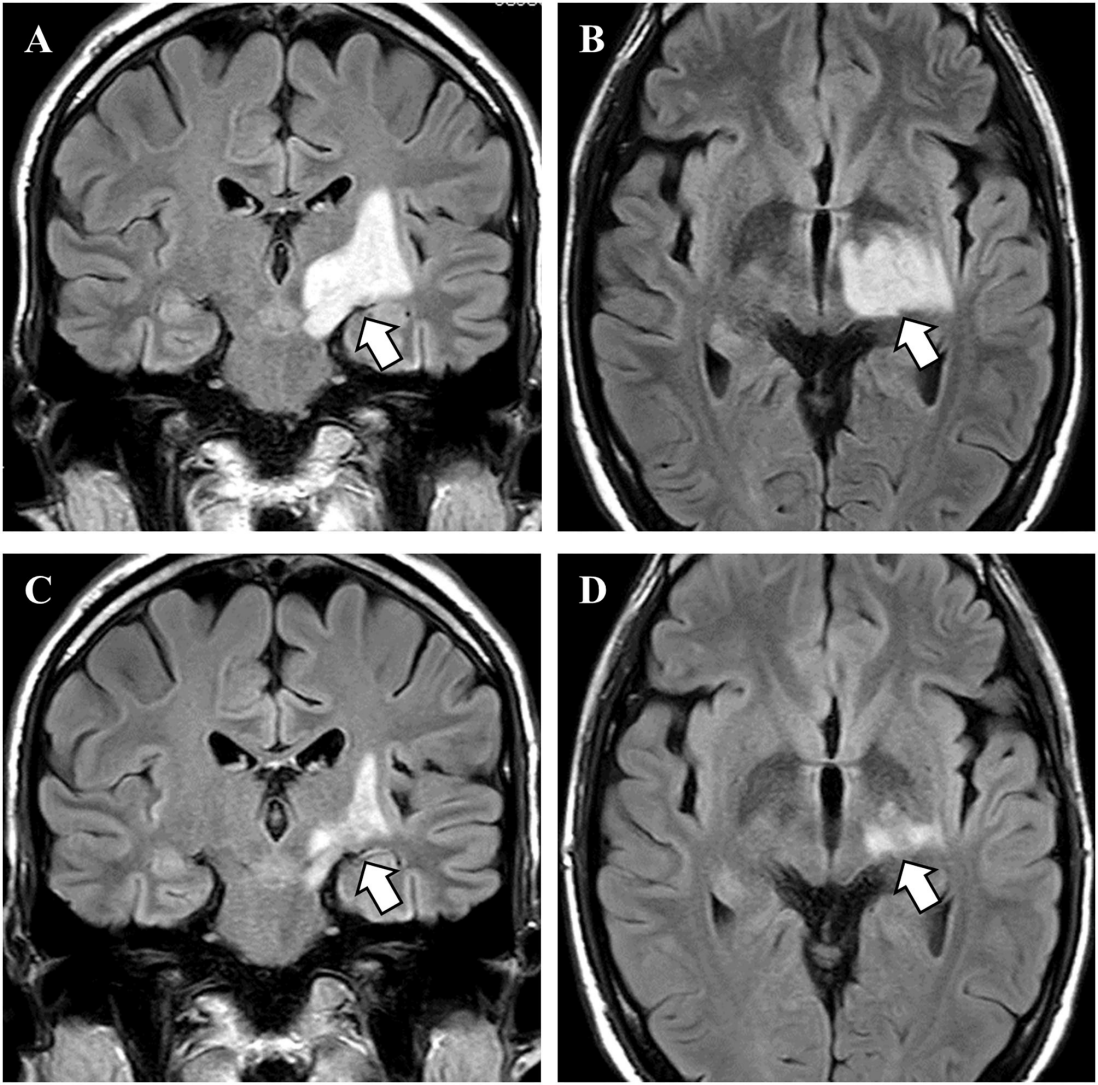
MRI findings before and after treatment. (A,B) Before treatment, brain magnetic resonance imaging showed a high-intensity lesion in the left basal ganglia (arrows). (C,D) After chemotherapy and immunotherapy, the brain lesion was reduced in size (arrows).
